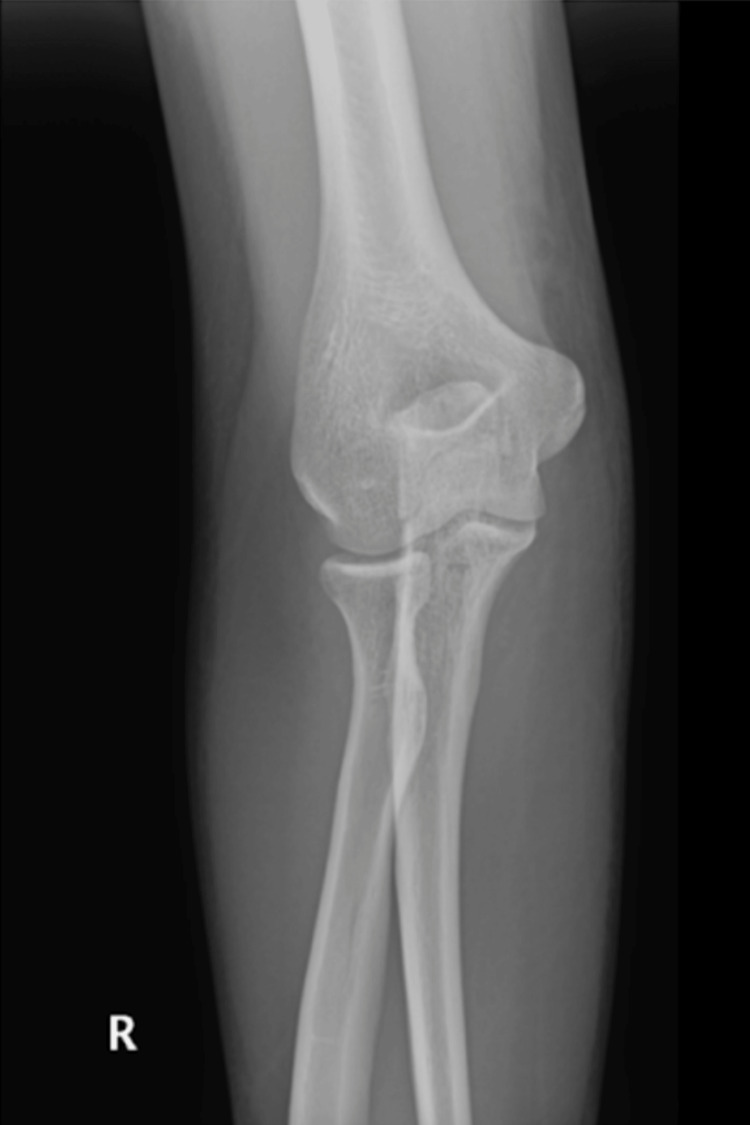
Six weeks postoperative AP X-ray image of the right elbow. AP, anteroposterior
